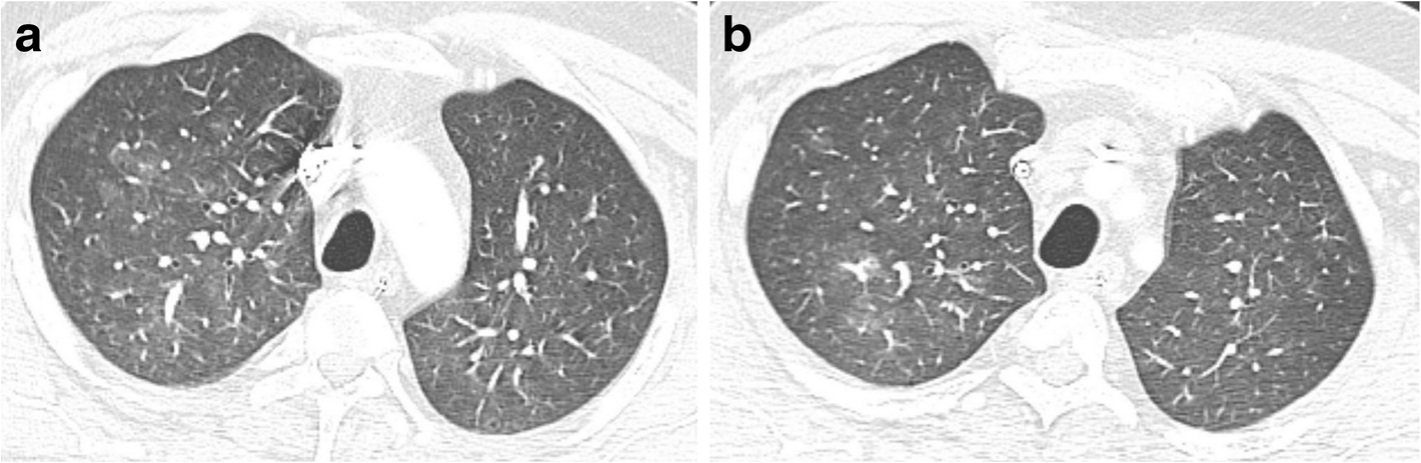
a, b CT- chest of COVID-19 patient shows widespread faint ground glass opacity upper lung lobe as well as reticular shadowing bilaterally
